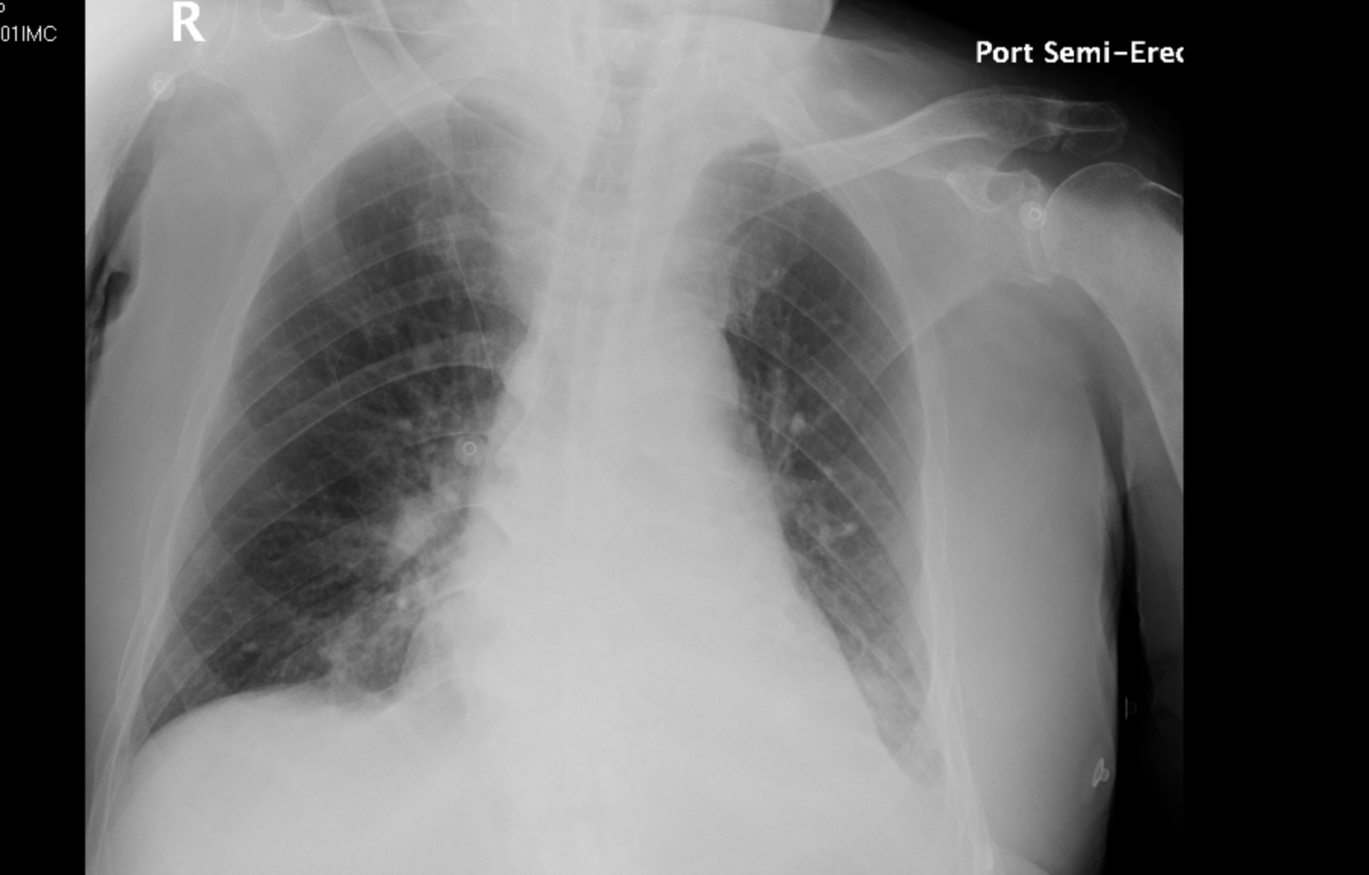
Case 3 chest X-ray on admission on April 12, 2020
